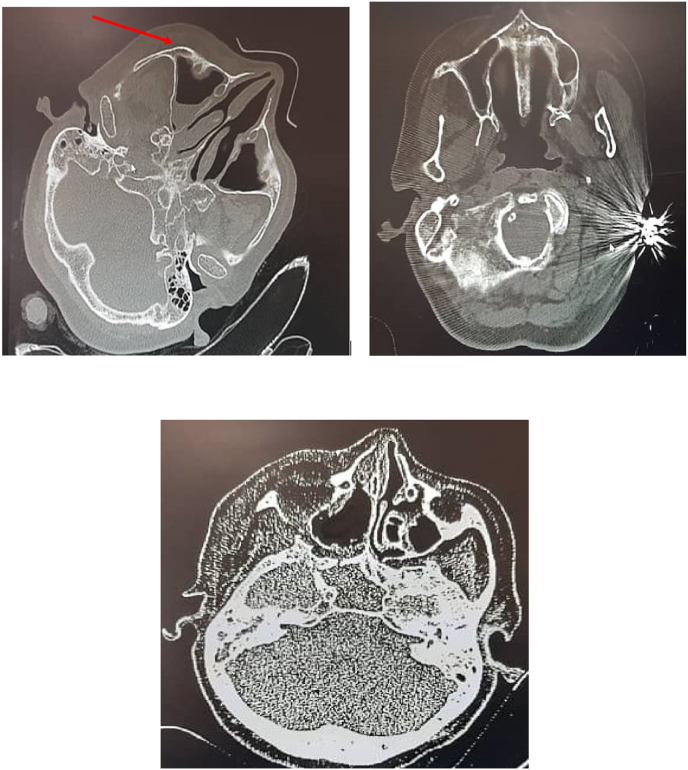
CT-scan of the brain, orbits, and paranasal sinuses.
